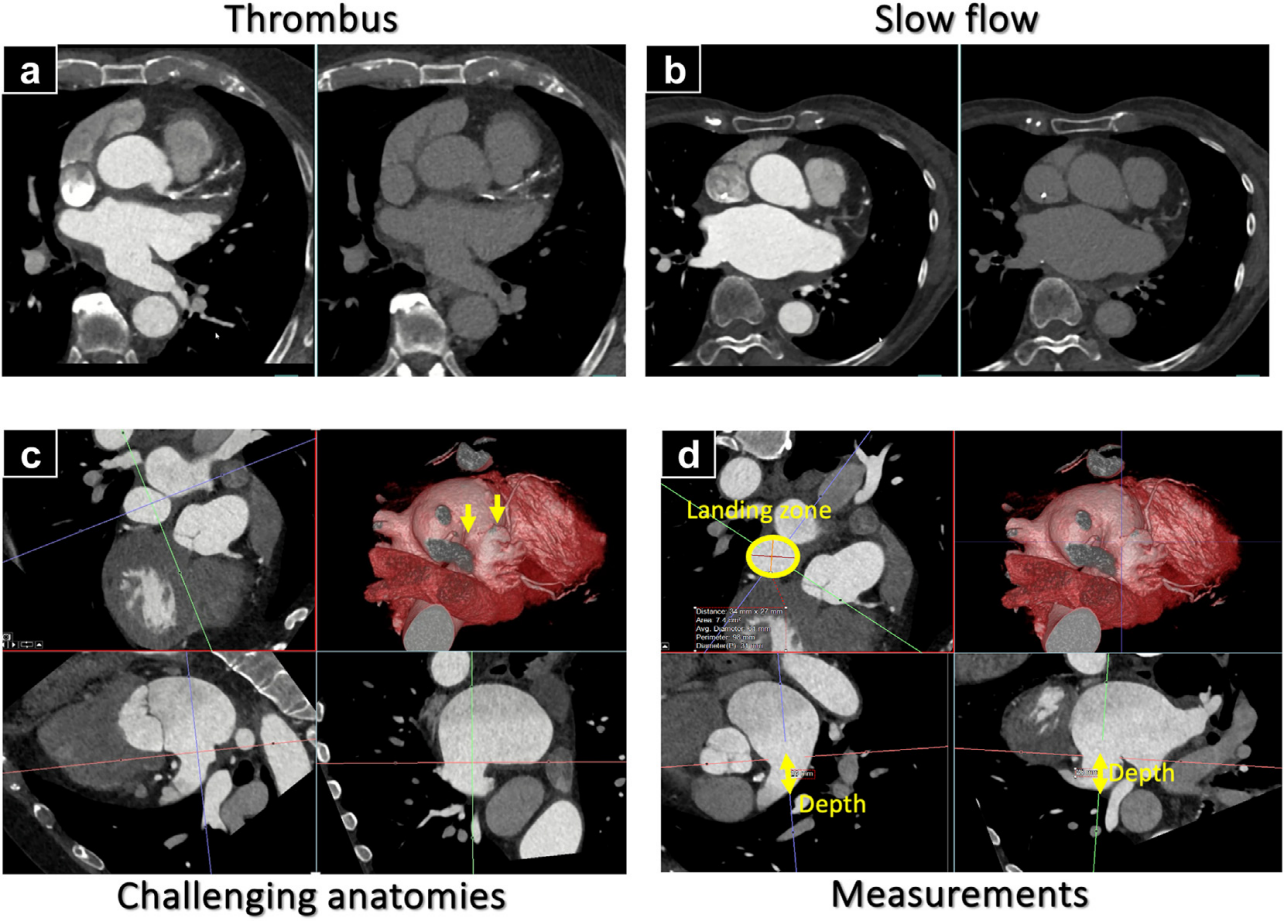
Common anatomic considerations for LAAO. (a) CT imaging of LAA thrombus; (b) slow flow in the LAA. Filling defect that persists with delayed acquisition is likely thrombus (panel A), but if it becomes homogenous, it is likely slow flow (panel B); (c) challenging anatomy with large LAA and multiple lobes; (d) note that given adequate implantation depth, LAAO was still successful in this patient. Videos of panels A-C are included in the supplement Abbreviations: CT, computed tomograph; LAA, left atrial appendage; LAAO, left atrial appendage occlusiony.
